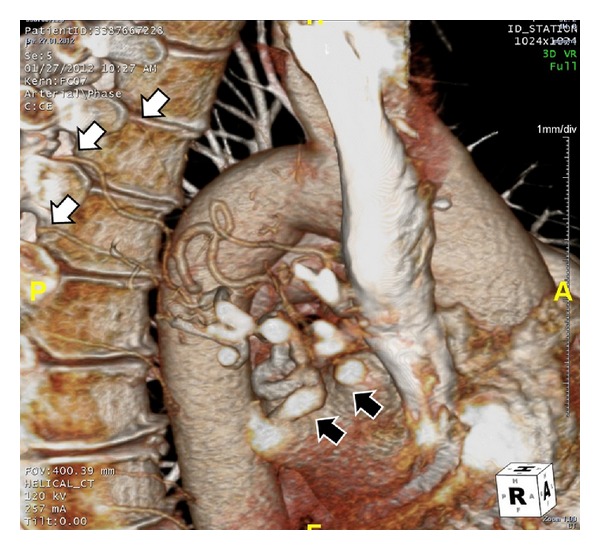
On 3D CT image, AVM was seen feeding from bronchial arteries (white arrows), draining to right pulmonary vein (black arrows).
